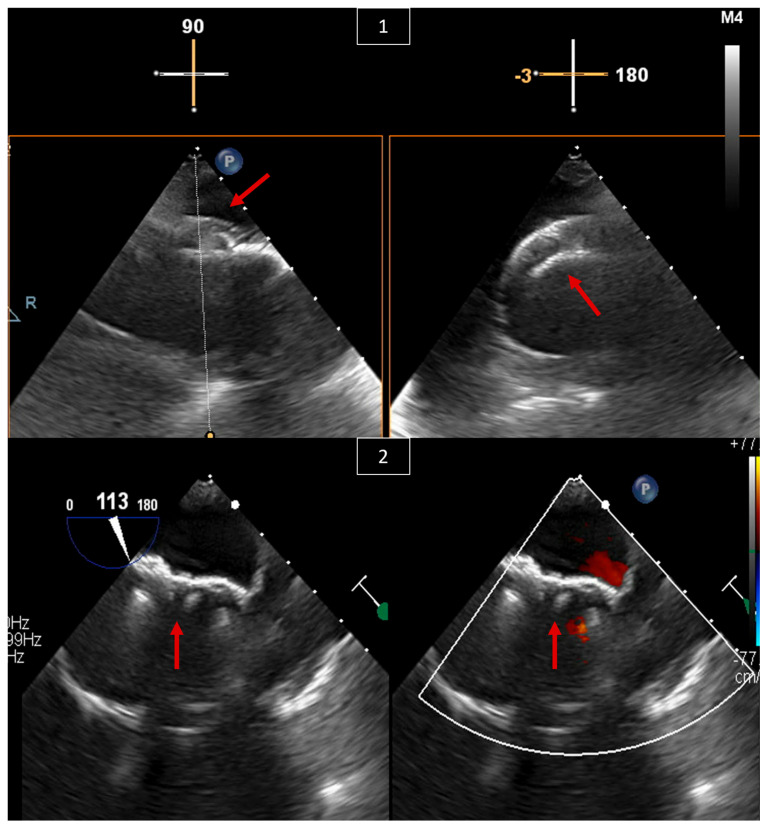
Fontan fenestration device closure in a 37-year-old male with lateral tunnel Fontan. (1) Mid esophageal view at 90 degrees with X-plane showing the fenestration closure device (red arrow) in stable position. (2) Showing the same device from (1) with and without color Doppler.
